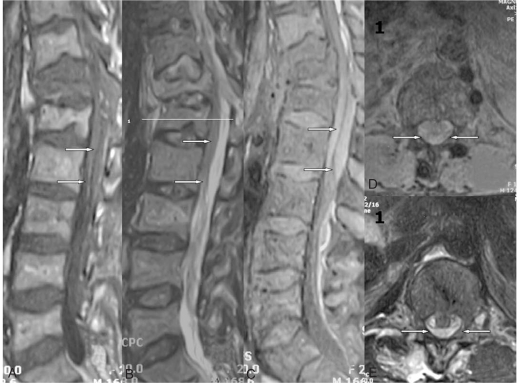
MRI findings on the initial trauma. Isotense on the T1-weighted image (A), hyperintense hematoma on the T2 sagittal and enhanced MRI (B, C), and hematoma compressing the cord on the T1 and T2 axial image (D, E).
